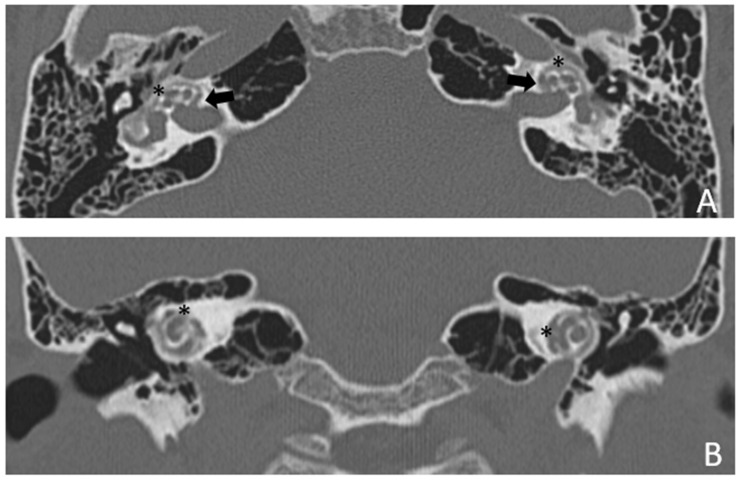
CT images of patient #2 with osteogenesis imperfecta. (A), axial plane; (B), coronal plane. A large pericochlear cavity is visible; arrows indicate its origin from the IAC, asterisks indicate the communication of the cavities with the cochlea.
